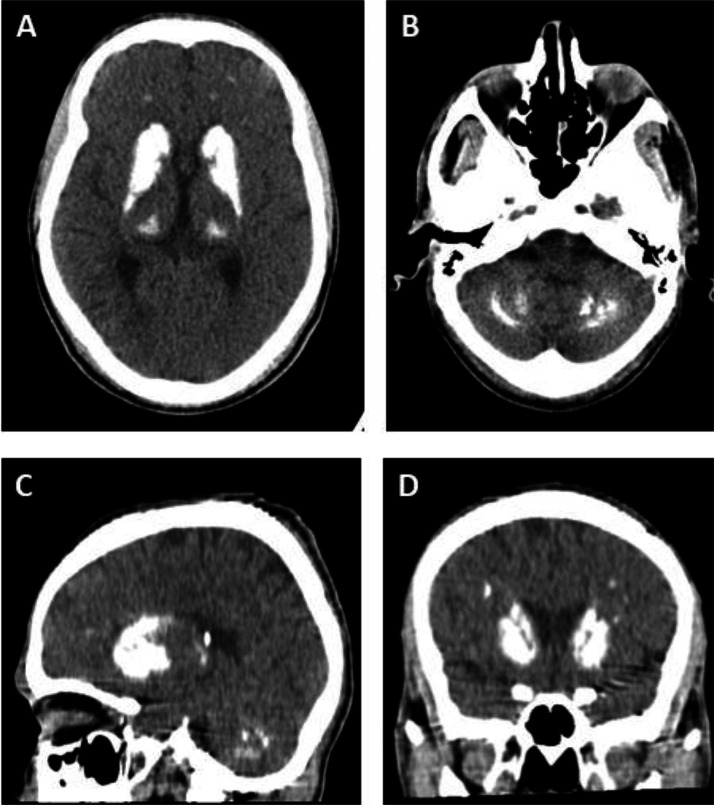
Brain computed tomography (CT) images reveal bilateral and symmetrical calcifications in key subcortical regions. (A) Axial CT scan showing bilateral calcifications in the basal ganglia. (B) Axial CT scan demonstrating symmetric calcifications in the dentate nuclei of the cerebellum. (C) Sagittal CT view highlighting calcified deep brain structures. (D) Coronal CT view confirming symmetrical calcium deposition across basal ganglia and cerebellar regions. These findings reflect abnormal calcium accumulation in areas essential for motor regulation and cerebellar coordination.
